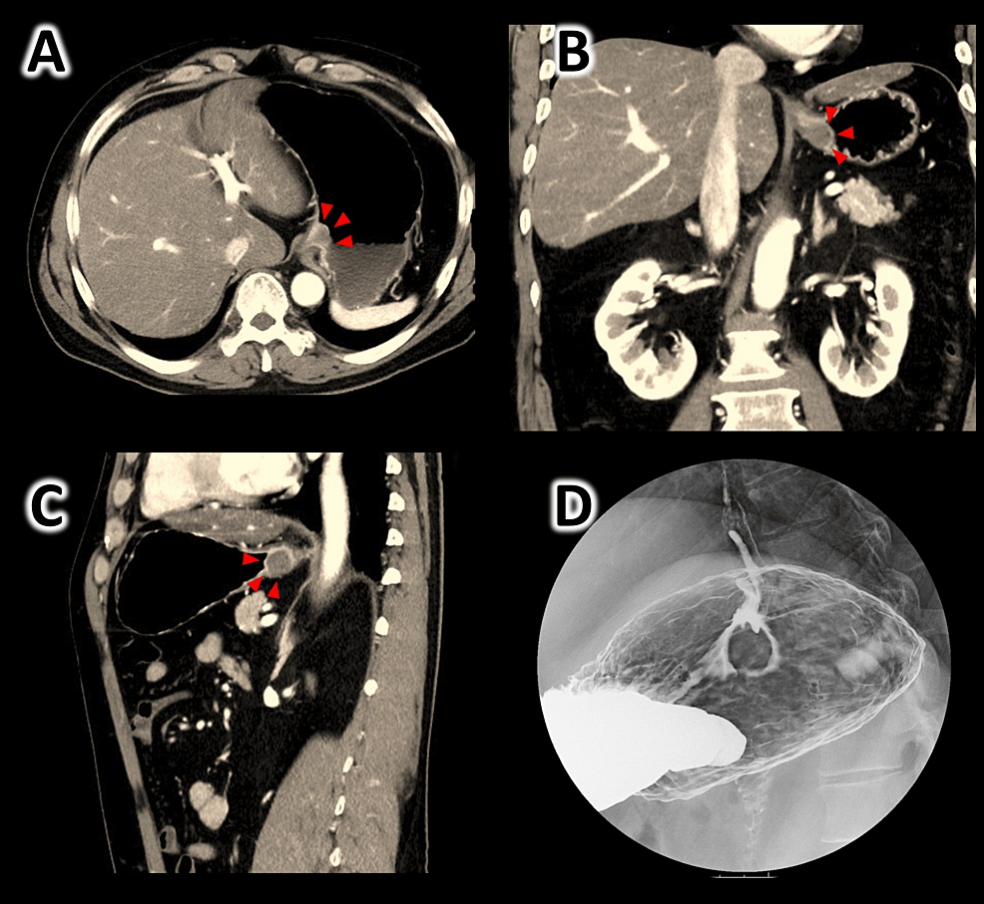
Preoperative thoracoabdominal contrast-enhanced CT image and X-ray image Red arrowheads show the lesion. (A) CT image, horizontal section. (B) CT image, coronal section. (C) CT image, sagittal section. (D) Contrast-enhanced X-ray image shows a 2-cm mass lesion just below the esophagogastric junction.
